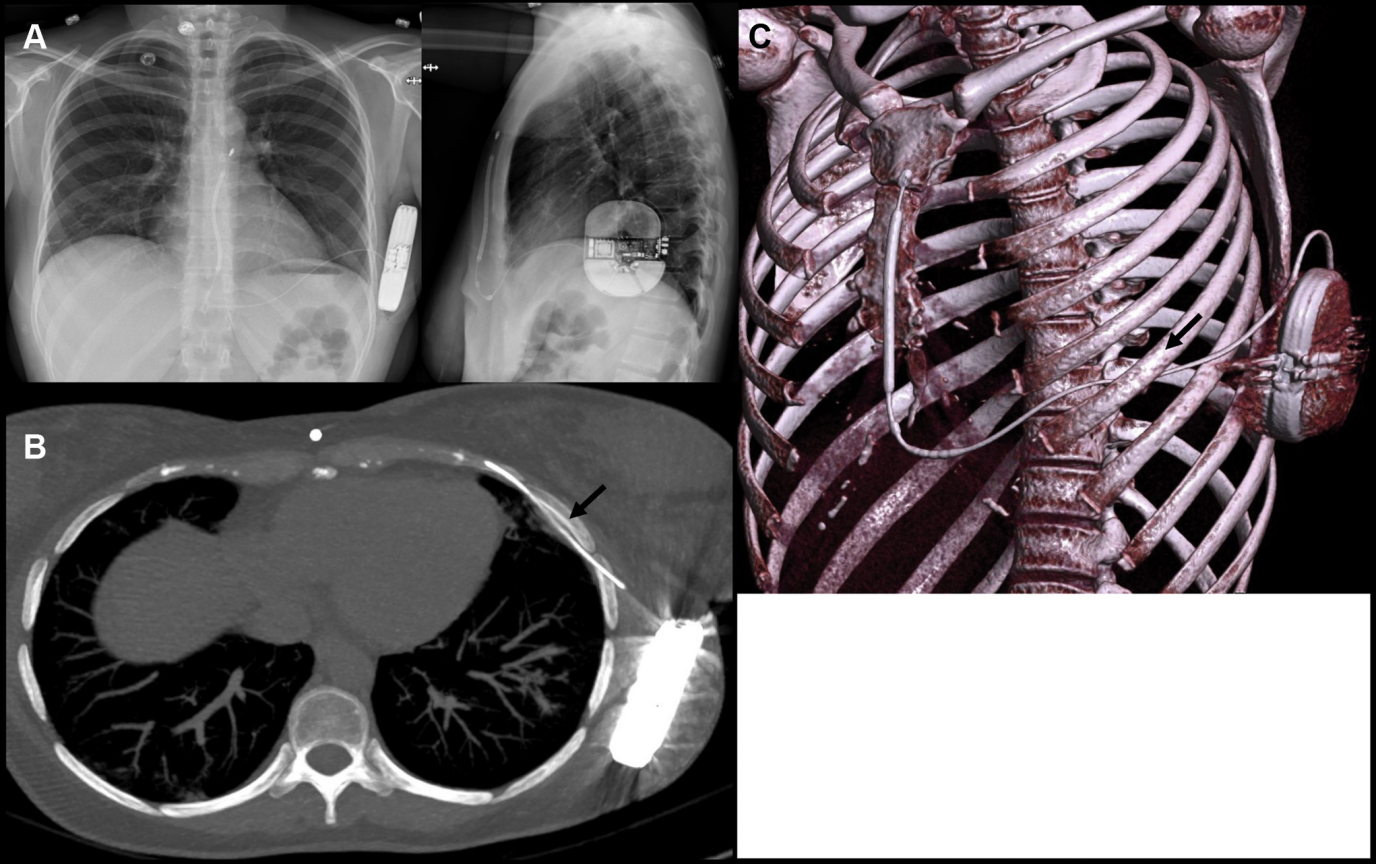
A: Chest radiograph after initial subcutaneous implantable cardioverter-defibrillator (S-ICD) implantation. B, C: Chest computed tomography with maximum-intensity projection (B) and volume rendering (C) showing intrathoracic trajectory of S-ICD lead (*black arrows*).
